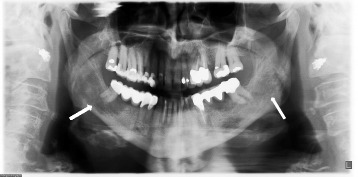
Panoramic X-ray. The arrows on the panoramic X-ray show osteolytic zones on the right and left angle of the lower jaw
